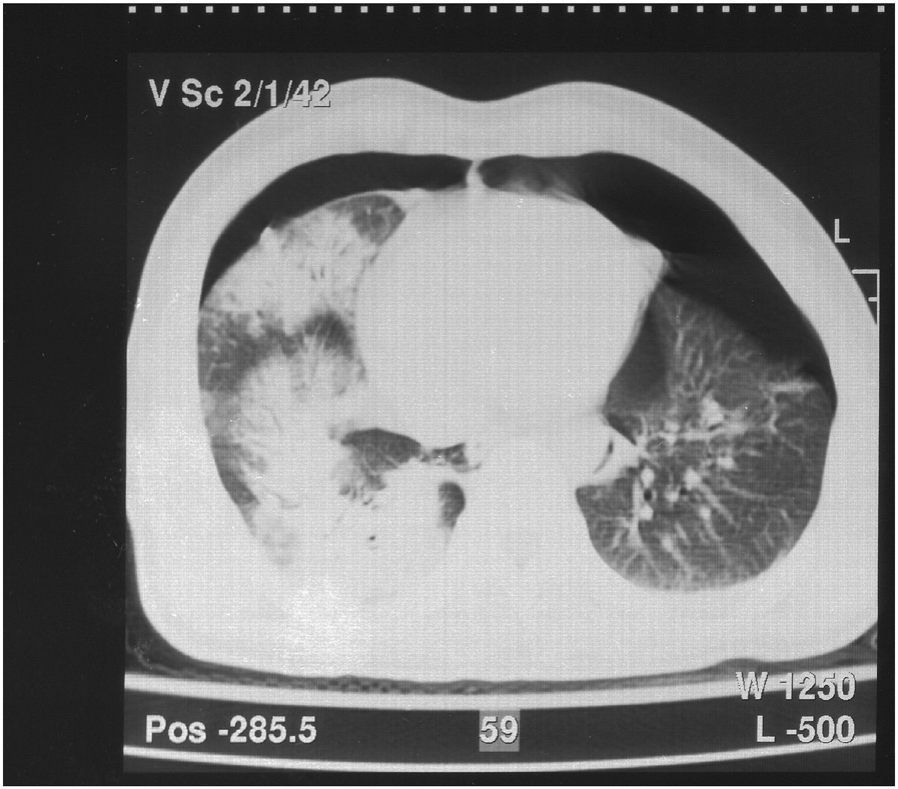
Computed tomography scan of the chest showing bilateral hemopneumothorax and multiple lung contusions, especially on the right.
